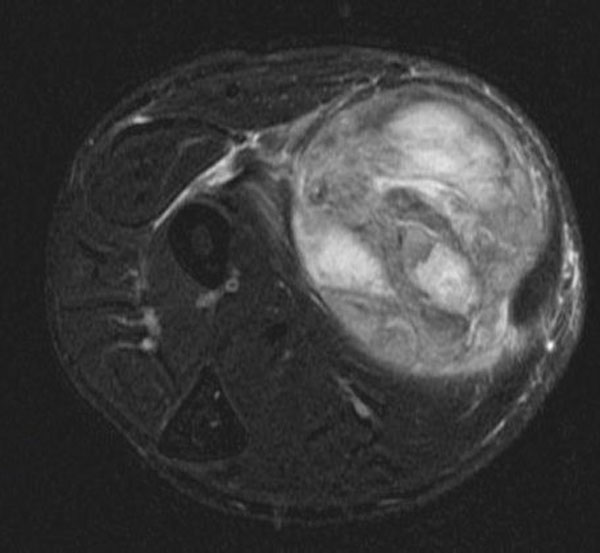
Axial T2-weighted magnetic resonance image with fat saturation of the left forearm showed a well defined, highly inhomogeneous tumour with liquid- and fat-equivalent parts displacing the surrounding tissue.
